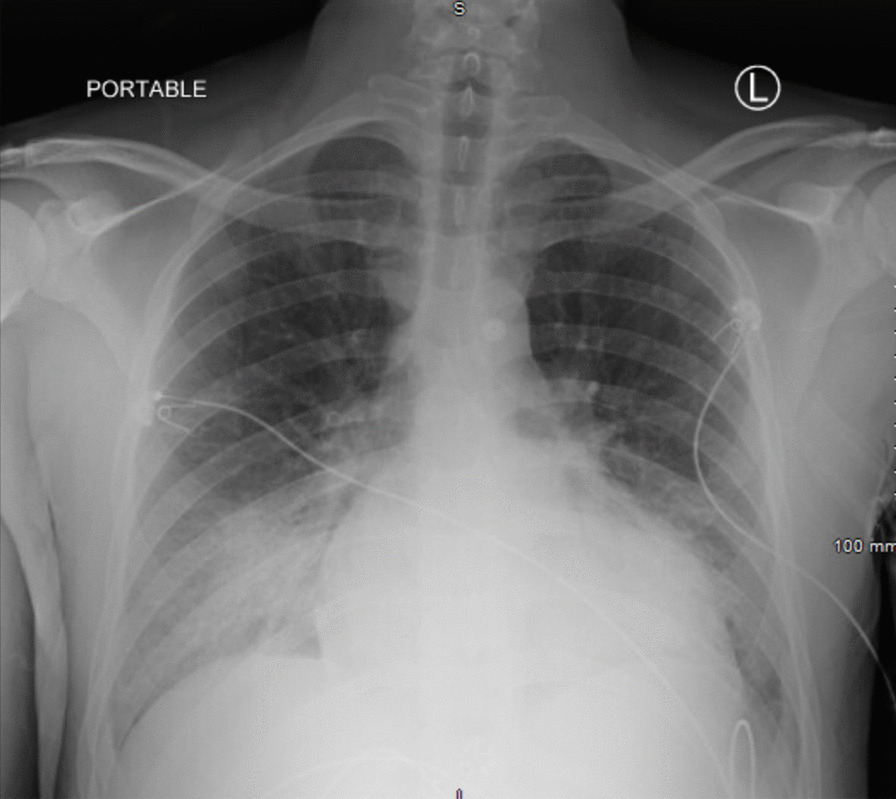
Chest radiograph. Hazy opacification of the right lower lung field and left lower lobe opacity. Cardiac silhouette is borderline in size
